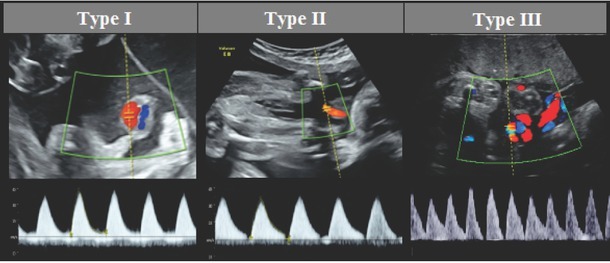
Ultrasound images of umbilical flow according to the classification of FGR in MC twins by Gratacós et al.^20^ FGR, foetal growth restriction; MC, monochorionic.
